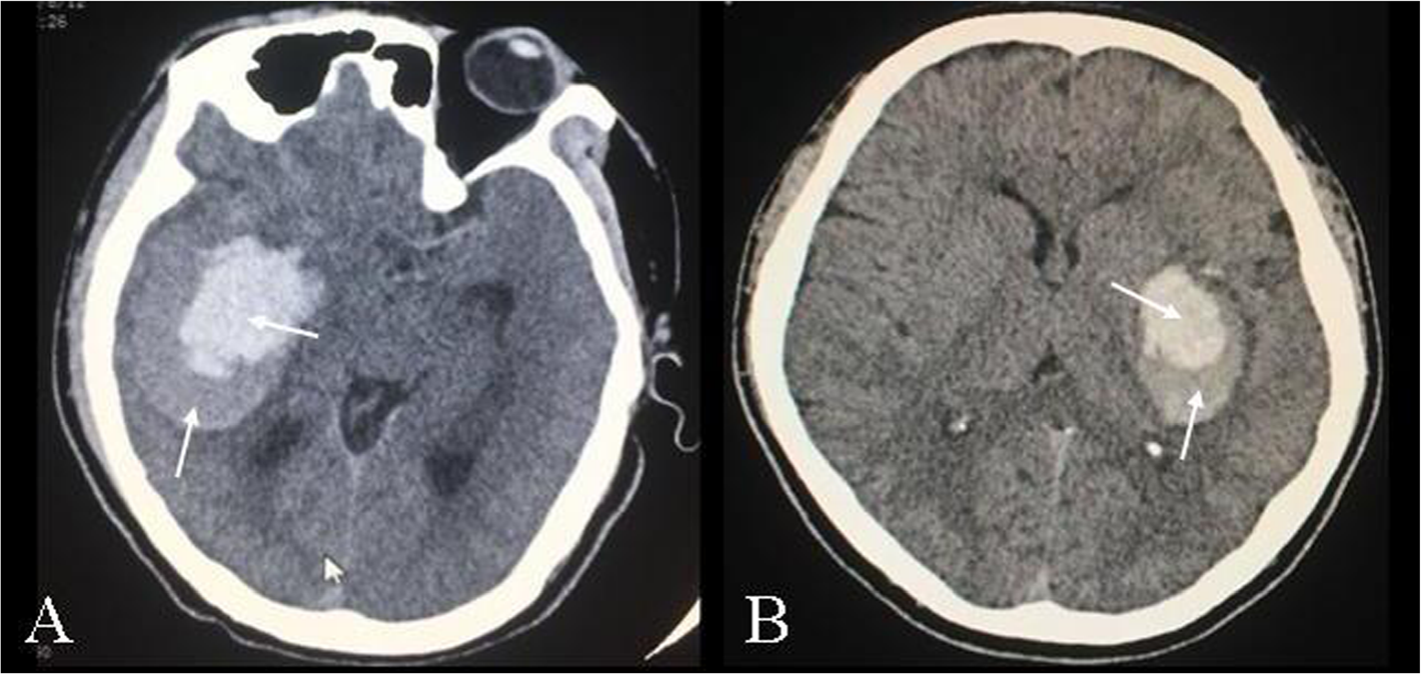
Blend signs on initial CT of patients with ICH. The ICH was located in the right (a) or the left (b) basal ganglia. The blend signs were composed of a hyperdensity and a relative hypodensity (pointed by the arrow). The boundary of the two parts was easily identified by naked eyes
